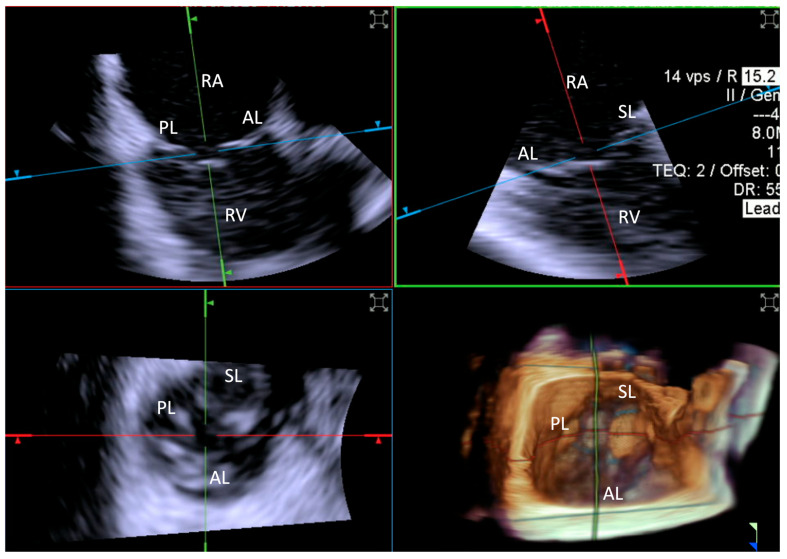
Home view. Home View refers to the primary imaging reference used with 4D-ICE throughout the T-TEER procedure, providing continuous monitoring of the tricuspid valve and device positioning. This view is generated through MPR, ensuring optimal visualization of anatomical structures and procedural steps. Initially, the evaluation is performed using 2D imaging without and with color Doppler, allowing for the assessment of leaflet motion and blood flow. The Home View remains the central imaging perspective during leaflet grasping, clip deployment, and post-implantation stability assessment, ensuring precise procedural guidance and real-time decision-making. Abbreviations: 4D-ICE: four-dimensional intracardiac echocardiography. AL: anterior leaflet. MPR: multiplanar reconstruction. PL: posterior leaflet. SL: septal leaflet. RA: right atrium. RV: Right Ventricle. T-TEER: tricuspid transcatheter edge-to-edge repair.
